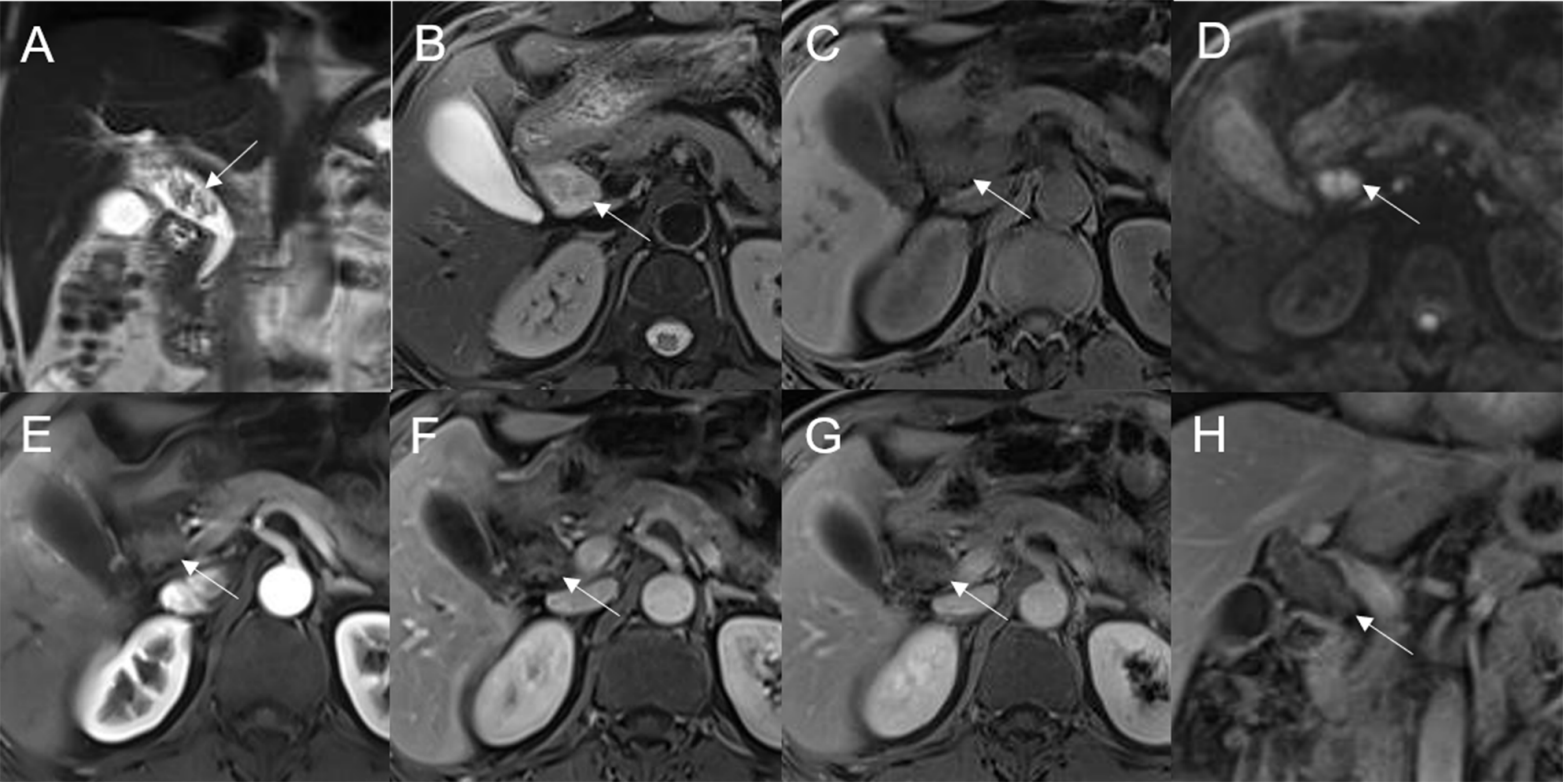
Adenoma in the common bile duct. The eccentric mass (white arrow) with a frond-like superficial appearance shows hyperintensity on T2WI (A, B), hypointensity on T1WI (C), and hyperintensity on DWI (D). The bile duct upstream of the lesion and at the lesion is dilated on coronal T2WI (A). The T1-postcontrast arterial phase (E), T1-postcontrast portal phase (F), and T1-postcontrast delayed phase (G, H) all show slight enhancement.
